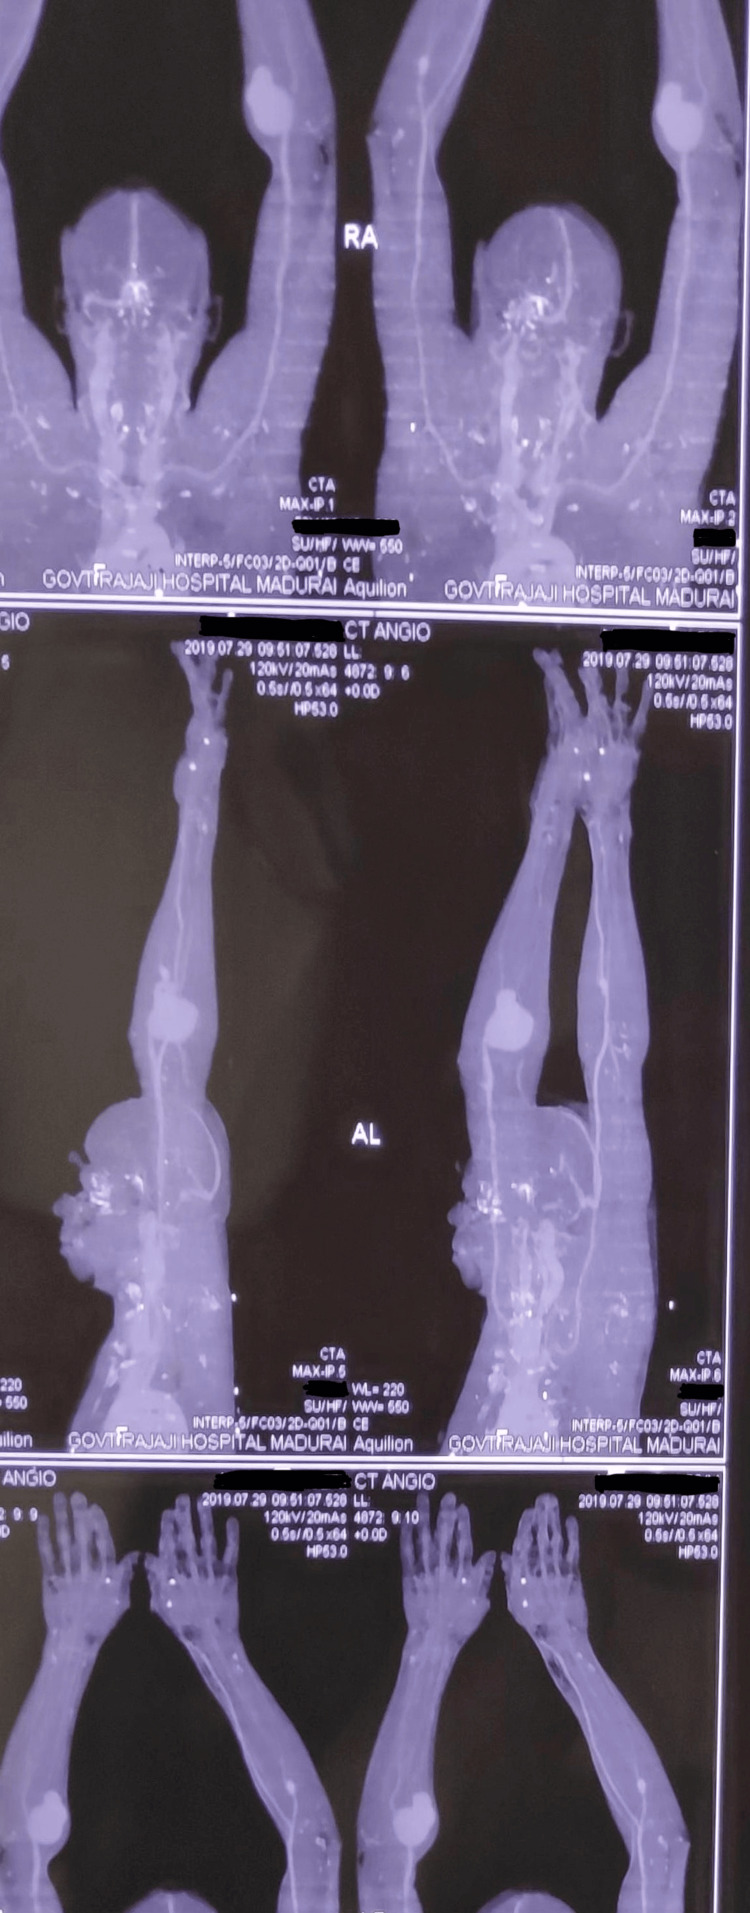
CT angiogram of the upper limb showing the left brachial artery aneurysm and right ulnar artery aneurysm
